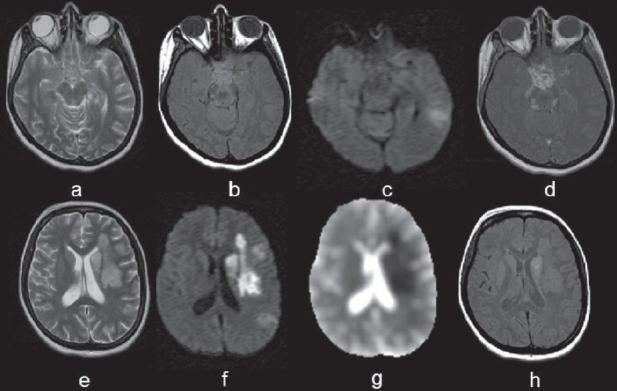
Tuberculous meningitis with vasculitis. T2W image (a) shows no apparent signal abnormality in the basal brain parenchyma. The corresponding magnetization transfer T1W image (b) shows hyperintensity in the perimesencephalic and supreseller cisterns. On DWI (c) restriction is noted in the left temporal region. The postcontrast T1W image (d) shows abnormal enhancement along the perimesencephalic and supraseller cisterns. In the same patient, axial sections at a higher level show hyperintensity on a T2W image (e) in the left middle cerebral artery (MCA) territory with hyperintensity on DWI (f) and low ADC values in the ADC map (g) suggesting a large left middle cerebral artery (MCA) territory infarct. The postcontrast T1W image (h) shows abnormal enhancement in the left caudate nucleus region
